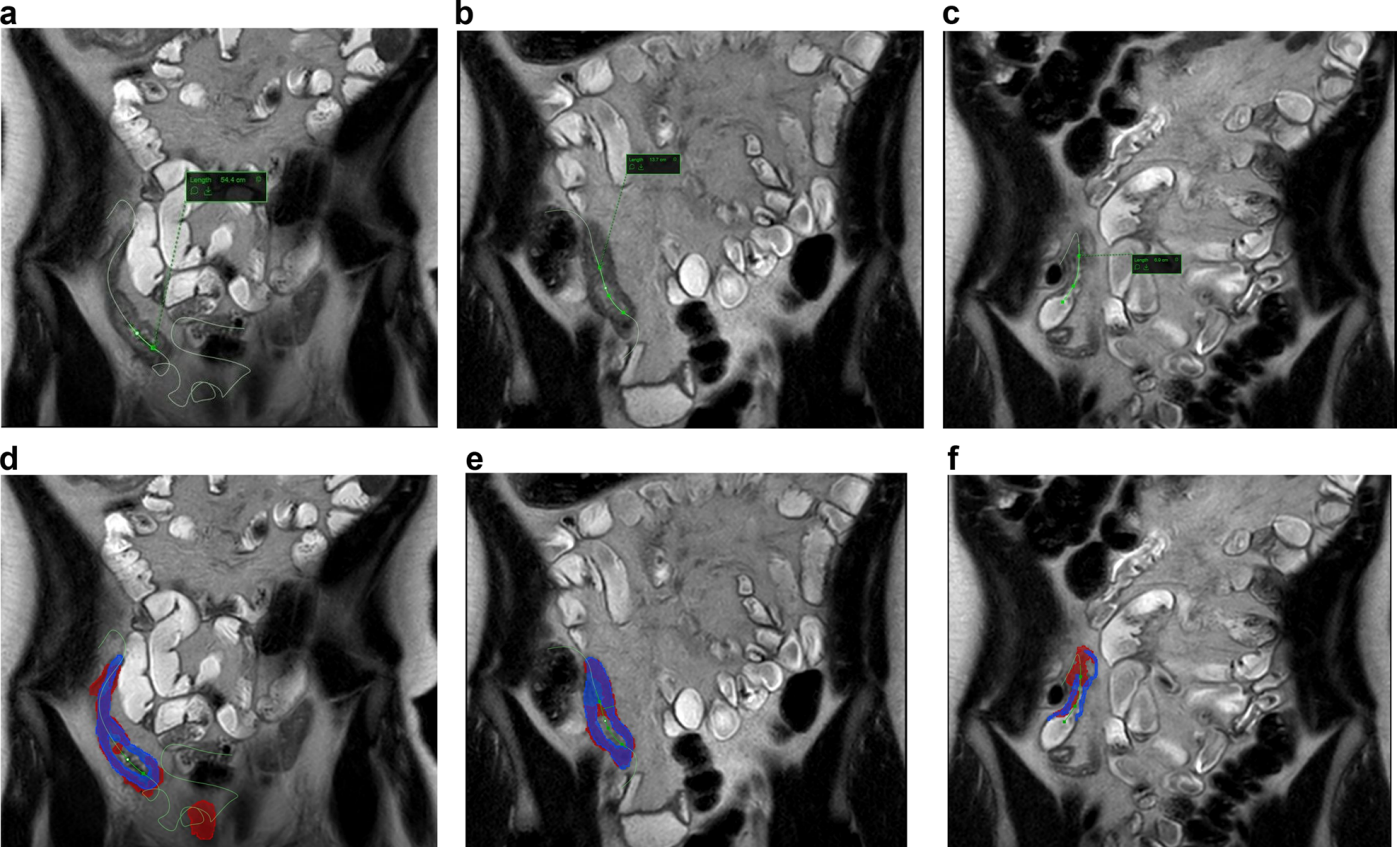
Eighteen-year-old male with newly diagnosed ileal Crohn disease. a–c Coronal single-shot fast spin-echo MR images at baseline (a), 6 weeks into medical treatment (b), and 6 months into medical treatment (c). Note the intraluminal curved green line demonstrating the length of disease called as centerline. The length of disease measured 54.4 cm (a), 13.7 cm (b), and 6.9 cm (c) at each time point, respectively. The manual segmentation is shown in blue color and semi-automated segmentation is shown in red color at baseline (d), 6 weeks into medical treatment (e), and 6 months into medical treatment (f)
